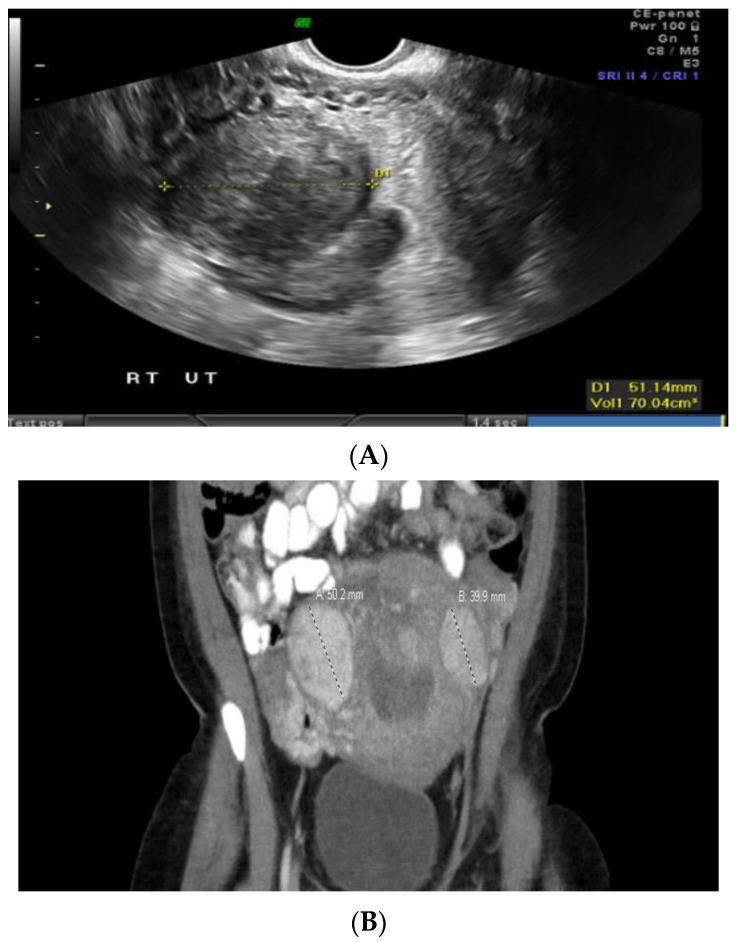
(A) Submucosal uterine fibroid. (B) Computed tomography (CT) scan-coronal section abdomen.
